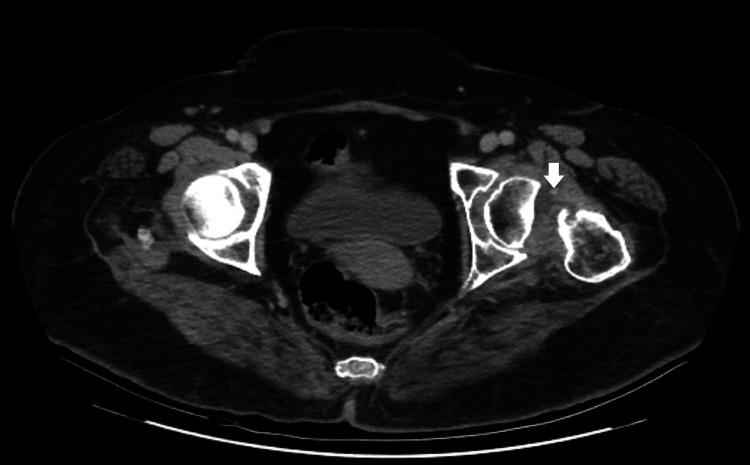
A non-contrast CT scan of the left hip in the axial section showing the lesion
